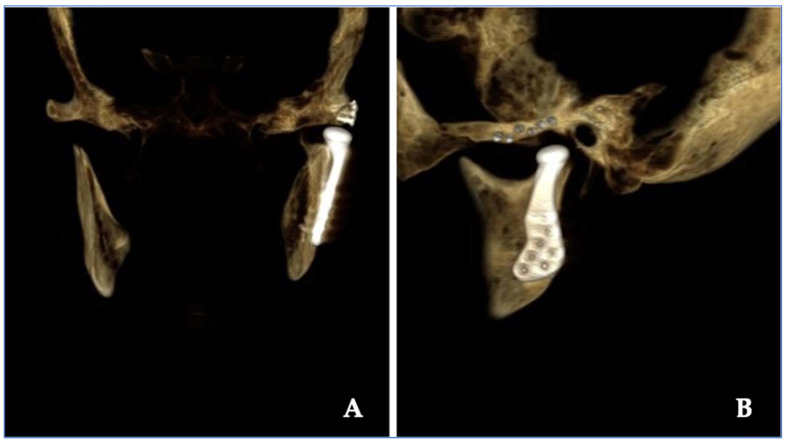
Postoperative tridimensional computed tomography reconstruction of clinical case shown in Figure 3. (A) Coronal view. (B) Sagittal view.
